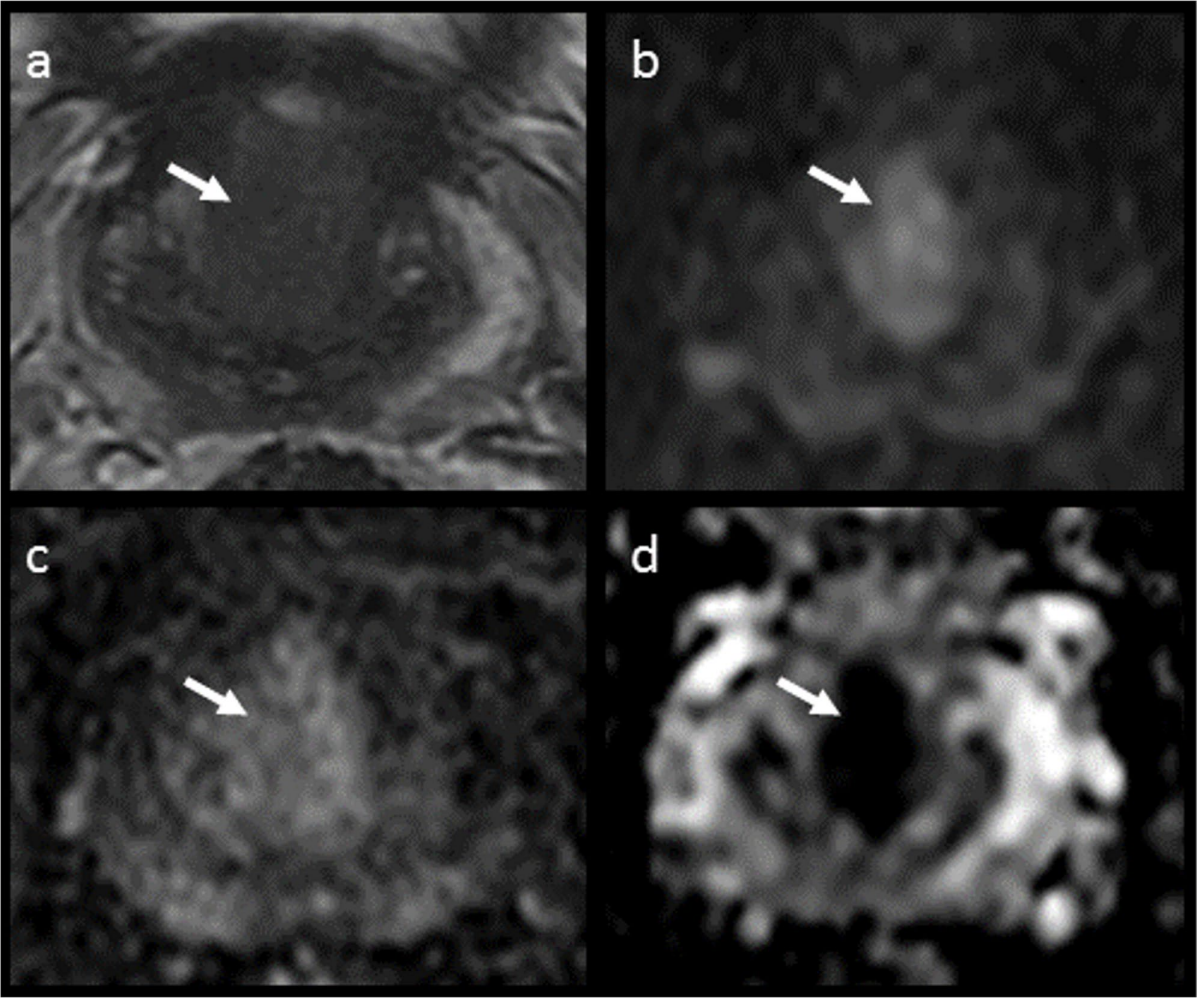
MRI of a 63-year-old patient originally reported as negative (PI-RADS 2). Elevated PSA levels (6.62 ng/mL) prompted a systematic biopsy despite MRI results, which revealed the presence of csPCa (Gleason score 3 + 4). At image retrospective review, a 20 mm hypointense lesion with obscure margins located at the base (midline) was identified in the T2-weighted sequence (white arrow, a), corresponding to a focus of restricted diffusion (white arrows, b and d) with associated contrast-enhancement (white arrow, c). These findings are in keeping with a PI-RADS 5 lesion, and the biopsy results matched the location of the MRI suspicious lesion
